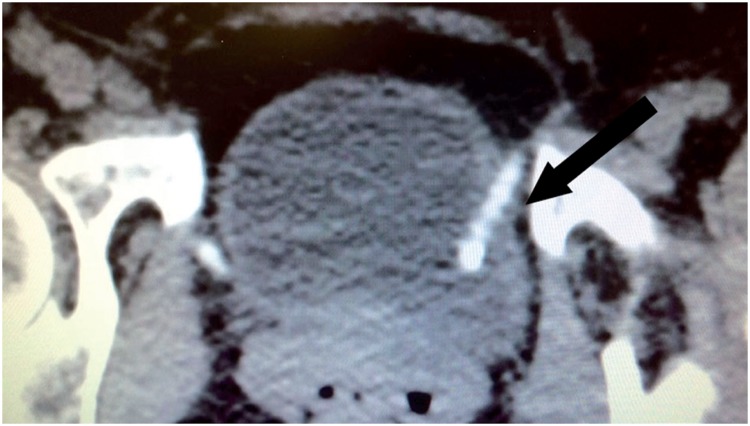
Pelvic CT scan shows calcified sling tape (arrow) eroding the bladder wall at the left side 2 years after a retropubic SSS.
